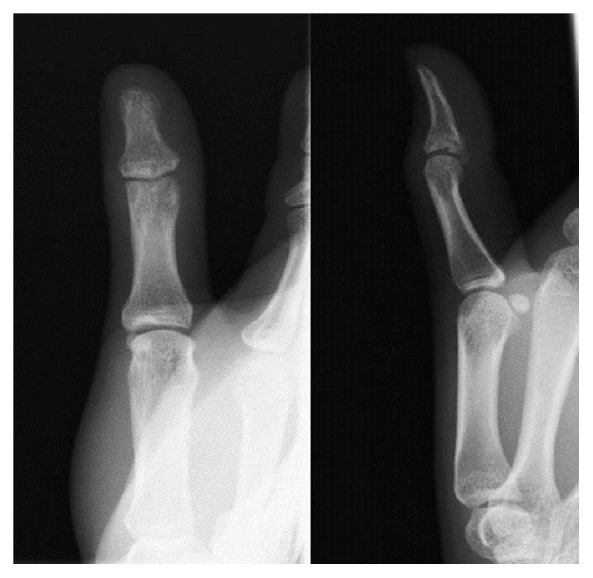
The plain radiograph at 3 months after surgery shows bony union of the avulsion fracture.
